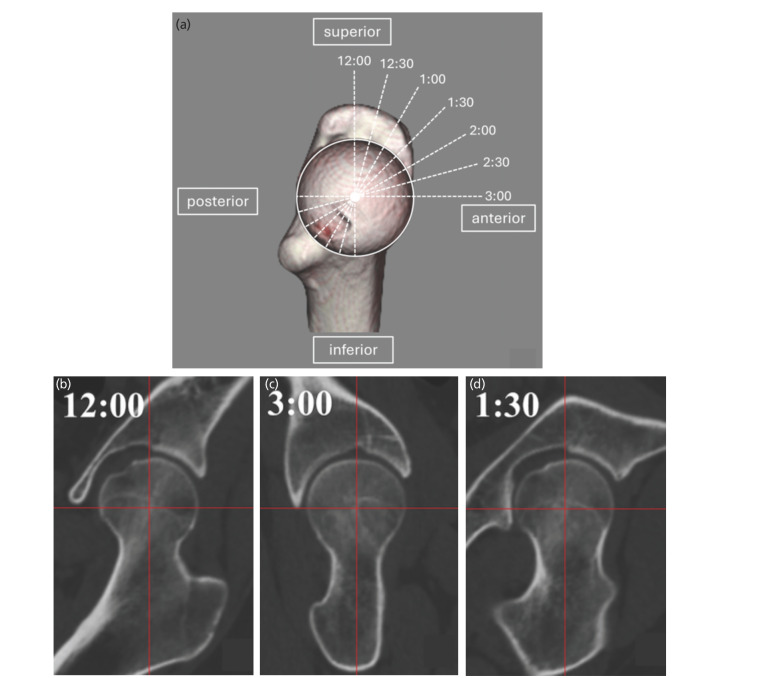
Computed tomography images of the left hip. (a) Seven increments perpendicular to the femoral neck axis were chosen for measurements of the alpha angles in each radial direction. (b) Coronal slices at the 12:00 clock position, (c) the 1:30 position, and (d) the 3:00 clock position.
